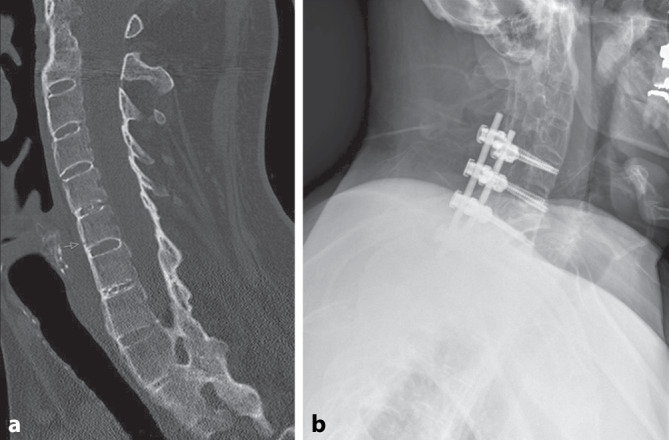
a Preoperative sagittal computed tomography (CT) image of the cervical spine demonstrating a Bechterew-associated B2 fracture of the C6 vertebral body according to the AO Spine classification. b Postoperative lateral radiograph showing dorsal instrumentation from C5 to Th1 following CT-navigated screw placement, confirming correct alignment and stable fixation
